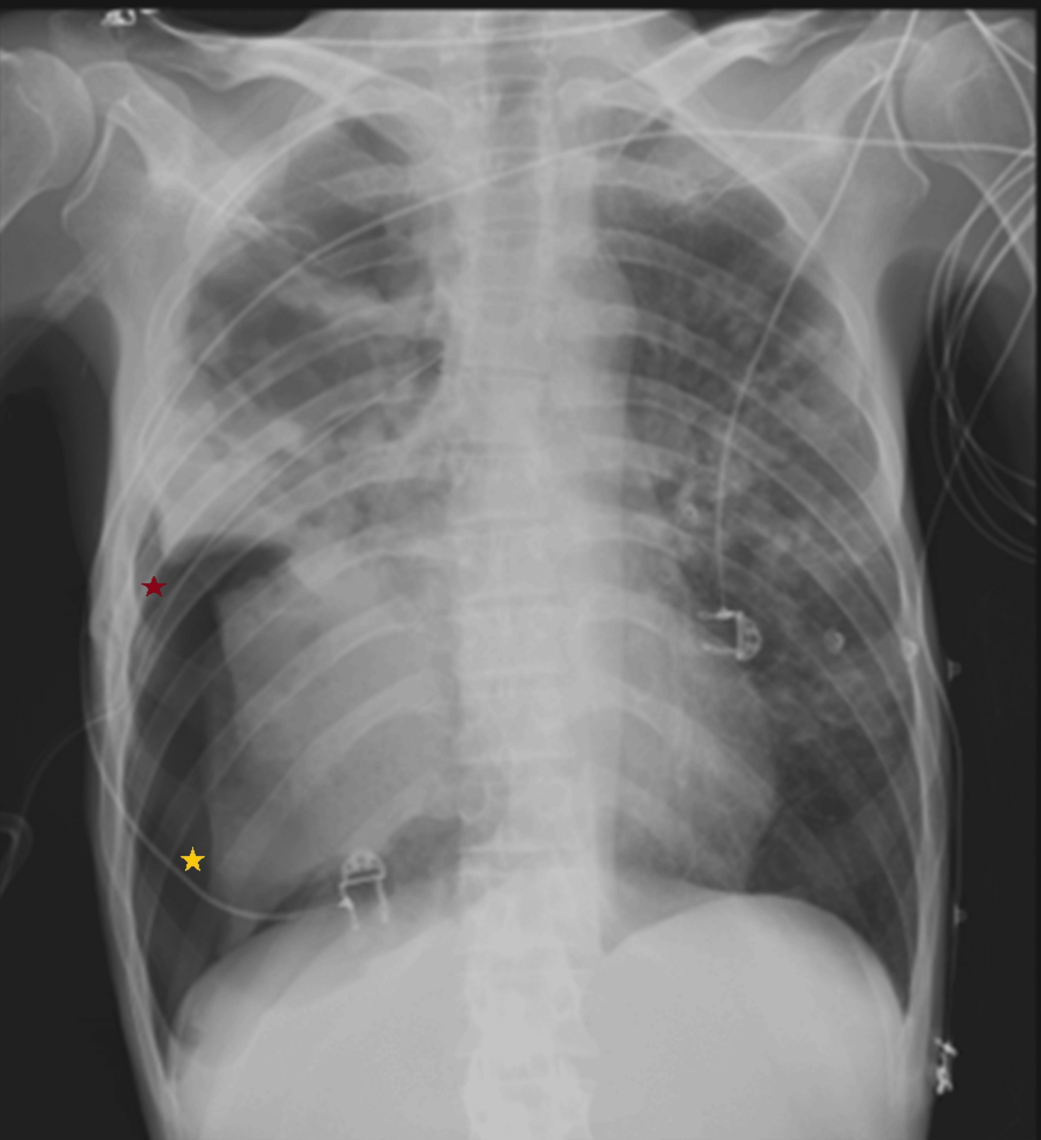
Chest radiograph following chest tube placement Emergency department chest radiograph after chest tube placement (red star), showing extensive bilateral lung opacities and a persistent right-sided pneumothorax (yellow star).
